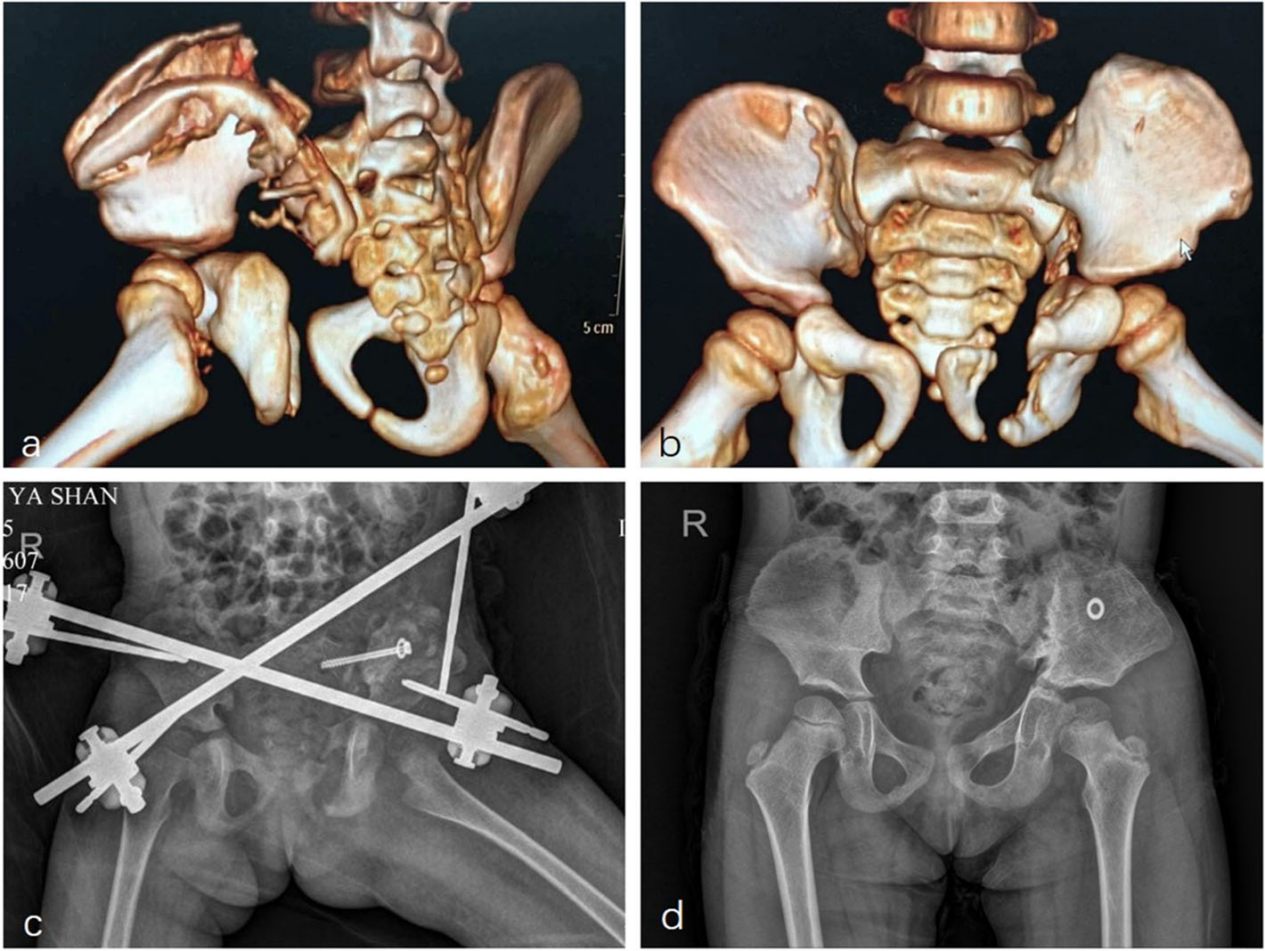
Case 2, a~b. Preoperative CT with 3 D reconstruction; c. postoperative X-ray; d. X-ray in the final follow-up
